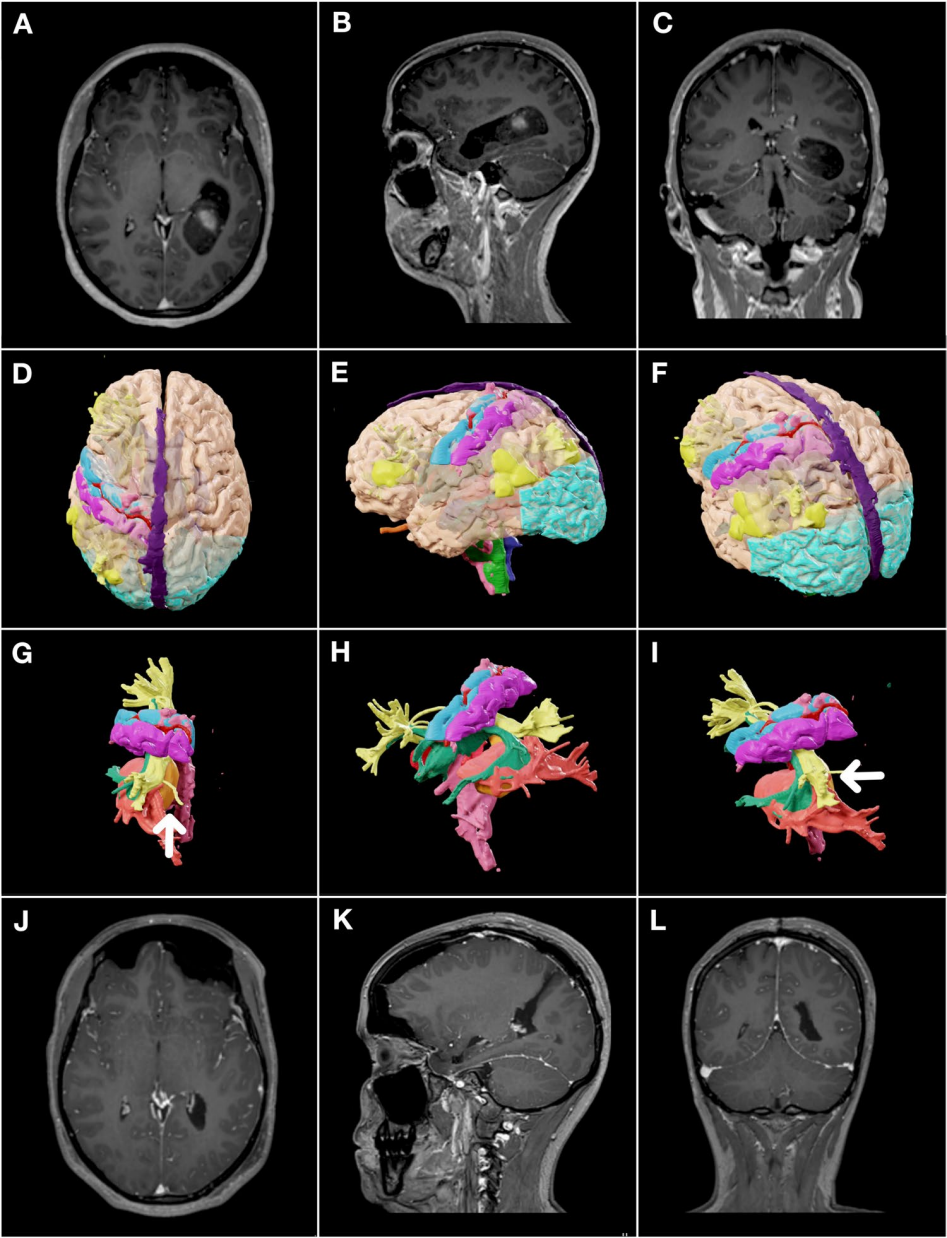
Peri-operative imaging and surgical planning. Contrast T1 MRI in the axial, sagittal and coronal planes pre (A-C) and post (J-L) operatively. D-I − 3D reconstruction of DTI fibre tracking in iPLanNet. Orange: lesion. Light red: optic radiation and lateral geniculate nucleus. Yellow: superior longitudinal fasciculus. Green: arcuate fasciculus. Light blue: precentral gyrus. Magenta: postcentral gyrus. Turquoise: occipital lobe. G - surgical line-of-sight view. H - sagittal view. I - Oblique view
